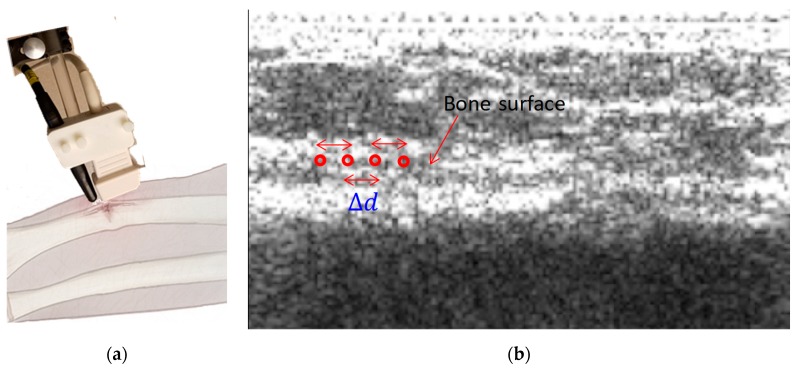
(a). Probe/hydrophone on the skin, where the fracture site is between the probe and hydrophone; (b) Conventional B-mode ultrasound-guided ultrasound with four radiation force excitation beam focuses (red circles) Δd apart. Time delay measurements are conducted between two excitations. The hydrophone is located at the left side of the image. The arrow shows the bone surface.
